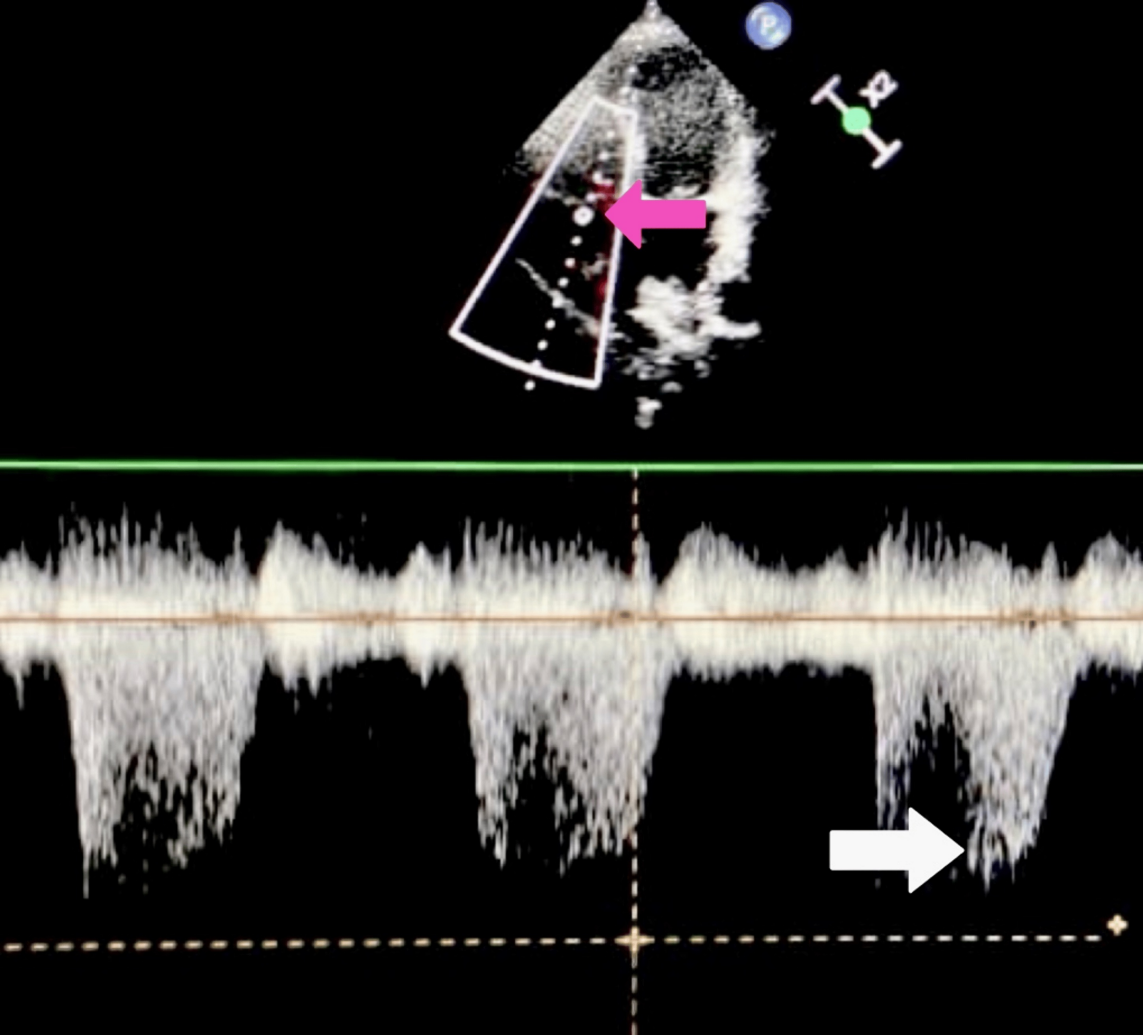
Continuous-wave Doppler tracing of tricuspid regurgitation from the apical four-chamber view. The dense, high-velocity jet envelope (white arrow) represents tricuspid regurgitant flow, indicating elevated right ventricular systolic pressure, suggestive of mild pulmonary hypertension. The pink arrow indicates flow across the tricuspid valve. These findings support the hyperdynamic circulatory state commonly seen in cirrhosis and hepatorenal syndrome
